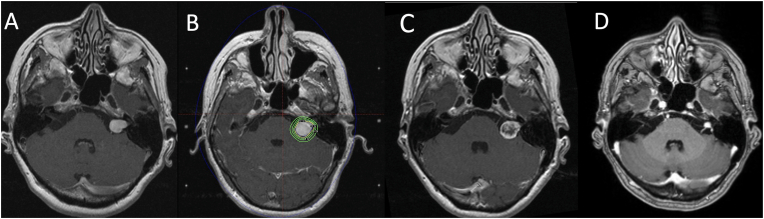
Radiological course and treatment of the vestibular schwannoma. Temporal progression from initial tumor diagnosis in 2003 to the most recent follow-up assessment of tumor control in 2019 on axial contrast-enhanced T1 weighted MR images. A) MRI obtained at the time of diagnosis in 2003 demonstrates an avidly enhancing lesion in the left cerebellopontine angle extending into the internal auditory canal, consistent with a Koos grade II vestibular schwannoma. B) MRI acquired for Gamma Knife® radiosurgery (GKRS) planning and dose plan in 2005 following tumor growth, with a cisternal component indenting the left cerebellar peduncle. C) Follow-up imaging in 2006 demonstrates central loss of enhancement, consistent with treatment-related necrosis. D) MR images obtained 14 years after GKRS shows a shrunken, avidly enhancing vestibular schwannoma in the left cerebellopontine angle.
